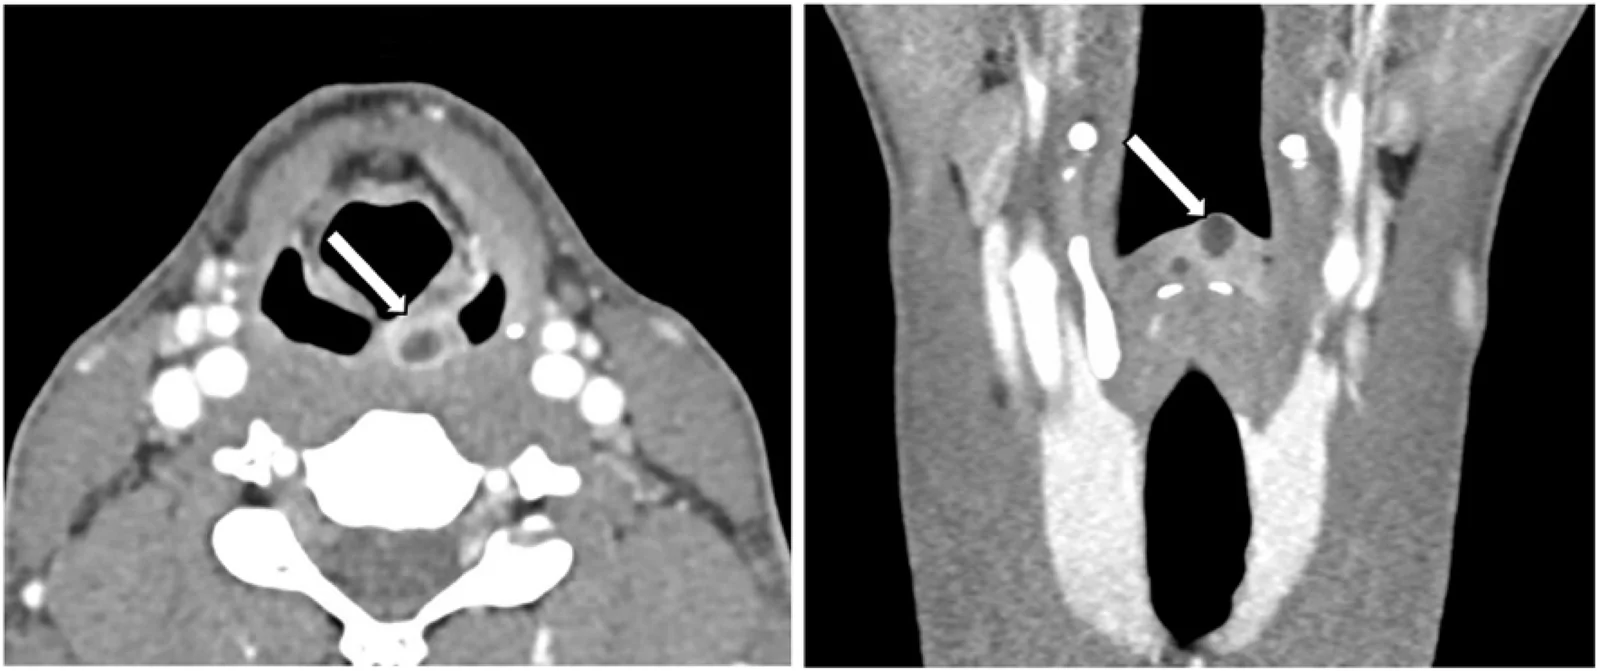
Axial and coronal post-contrast CT images at the time of second hospitalization show reduction of the aryepiglottic fold thickening, more evident on the right, with persistent enhancement on the left. A better-defined cystic lesion with peripheral enhancement is now seen along the inferomedial aspect of the left aryepiglottic fold (arrows).
